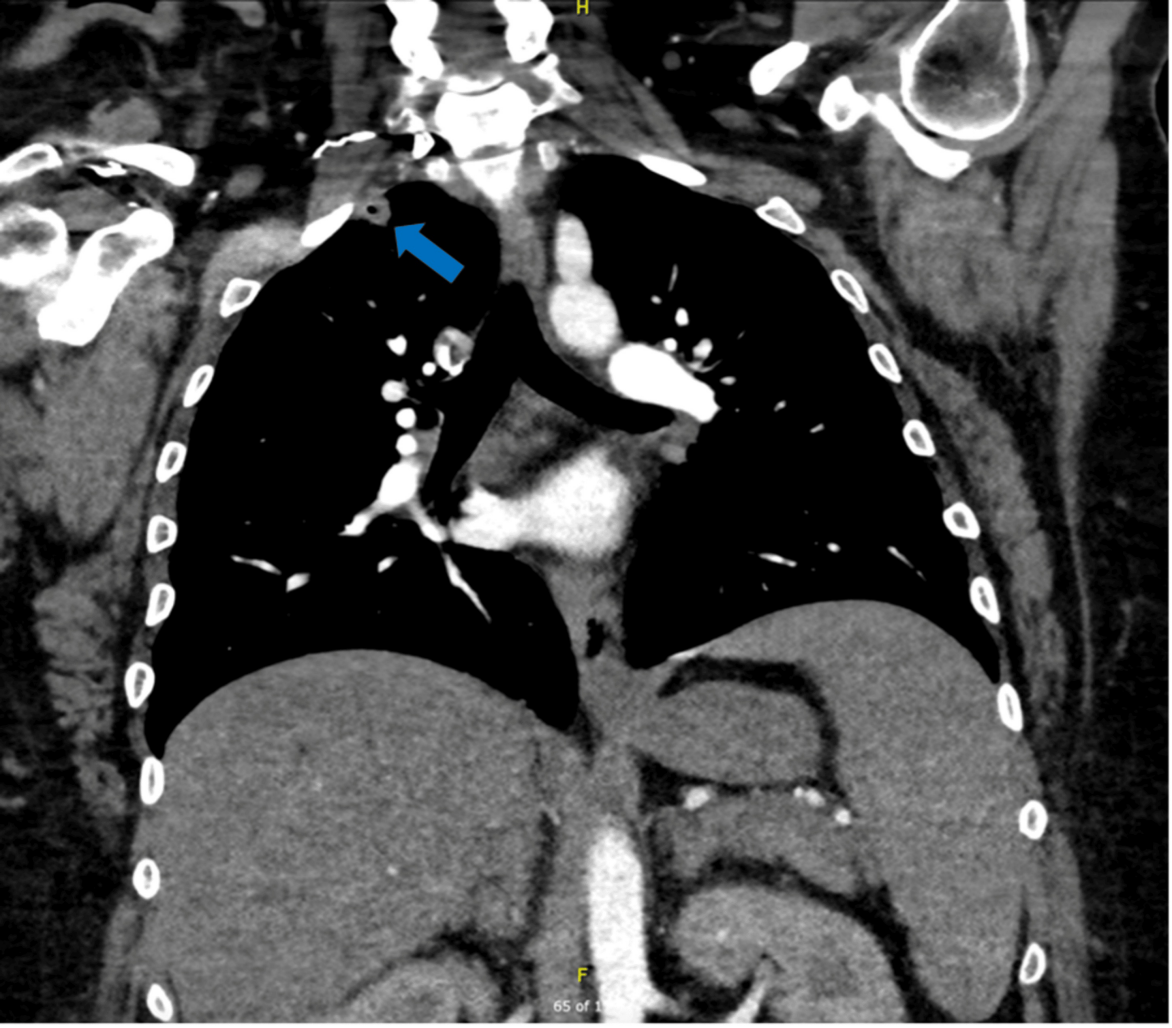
Computed tomography pulmonary angiography Coronal view demonstrating peripheral, wedge-shaped nodules concerning for septic pulmonary emboli (blue arrow).
